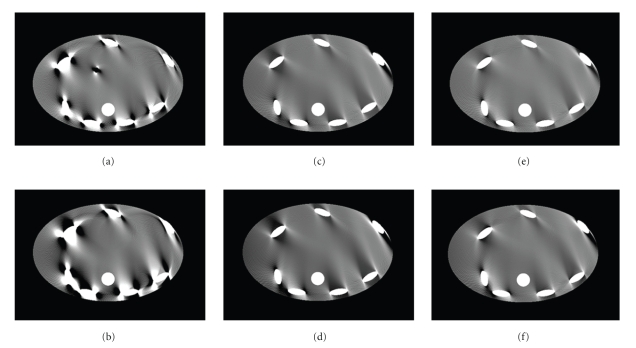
Worst-case images from (a) 3rd generation CT system with 40 mm coverage, (b) 3rd generation CT system with 80 mm coverage, reconstructed using FDK with Parker weighting approach, (c) multi-axial acquisition CT system with 120 mm coverage, (d) multi-axial acquisition CT system with 160 mm coverage, reconstructed with slice substitution approach using FDK parker weighting slices, (e) multi-axial acquisition CT system with 120 mm coverage, and (f) multi-axial acquisition CT system with 160 mm coverage, reconstructed with cone-angle dependent weighting reconstruction. Grayscale: (−50 HU, 50 HU). Note that the worst-case slices for 3rd generation system are the slices located at the edge of scan FOV, and the worst-case slices for multi-axial acquisition system are the slices located in between two sources.
